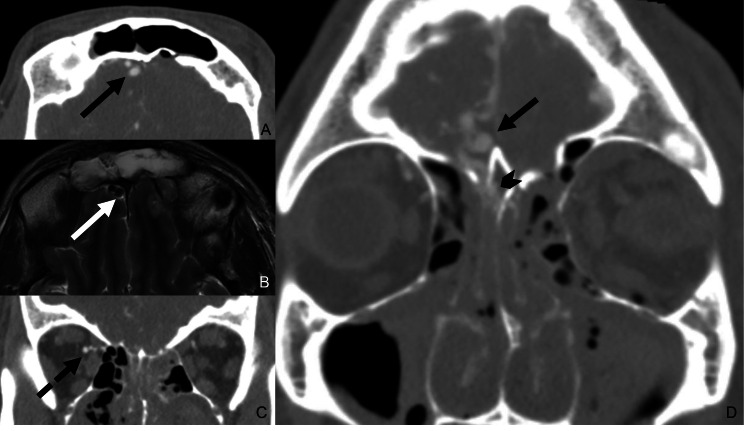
ACF-dAVF on CTA and MRI Axial (A) and coronal (C and D) CTA images demonstrates enlarged cortical veins along the right anterior cranial fossa (solid black arrow) and prominent right ophthalmic and ethmoidal arteries (dotted black arrow). The fistula point is centered at the fractured cribriform plate (black arrowhead). Axial T2 MRI sequence (B)  shows flow void of the enlarged cortical veins. ACF: anterior cranial fossa; dAVF: dural arteriovenous fistulas; CTA: computed tomography angiography
